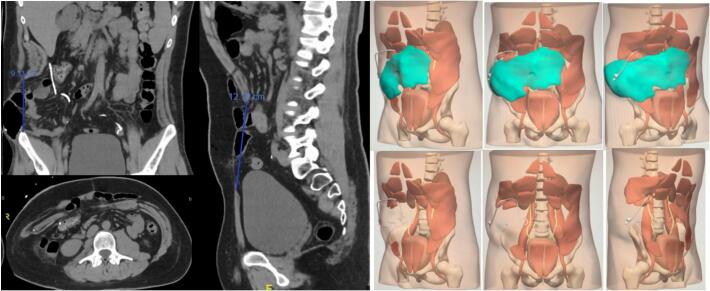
Abdominal CT scan six weeks after the administration of botulinum toxin and 3D reconstruction of the abdominal wall defect.
